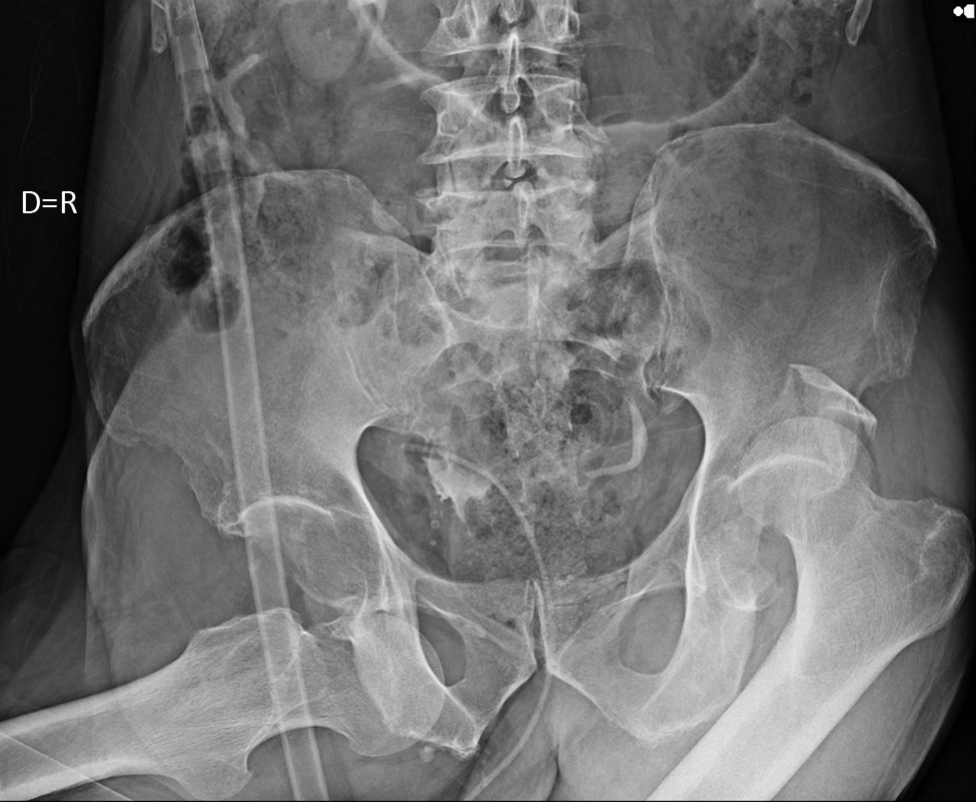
AP radiograph of the pelvis showing asymmetric bilateral dislocation of the hips.
